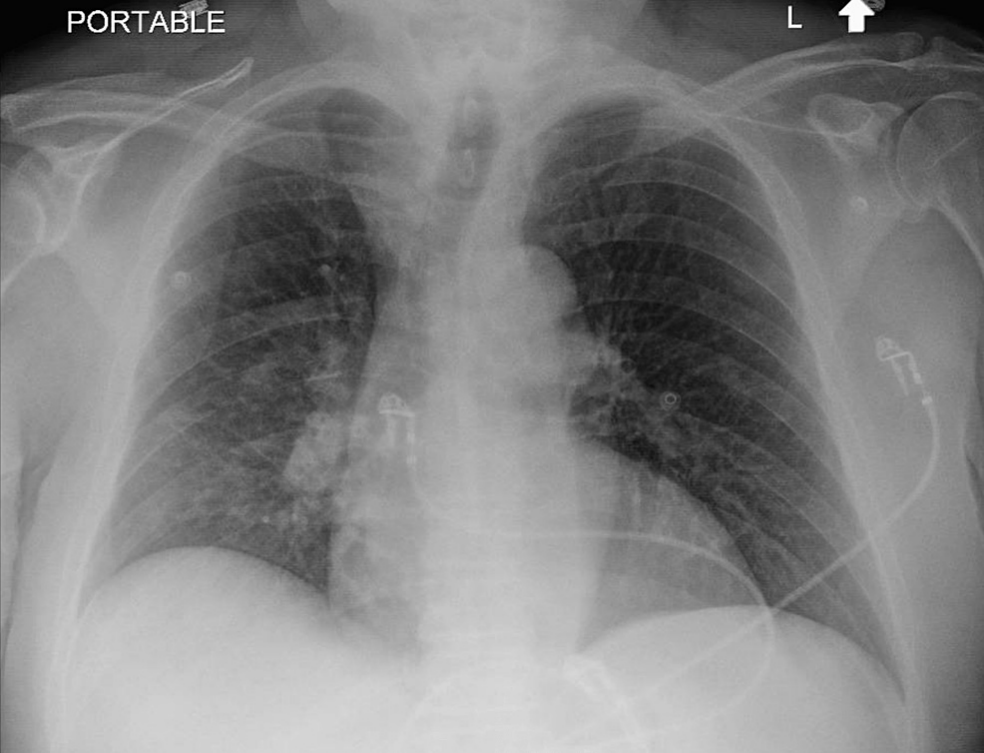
Chest X-ray shows evolving right lower lobe opacity.
